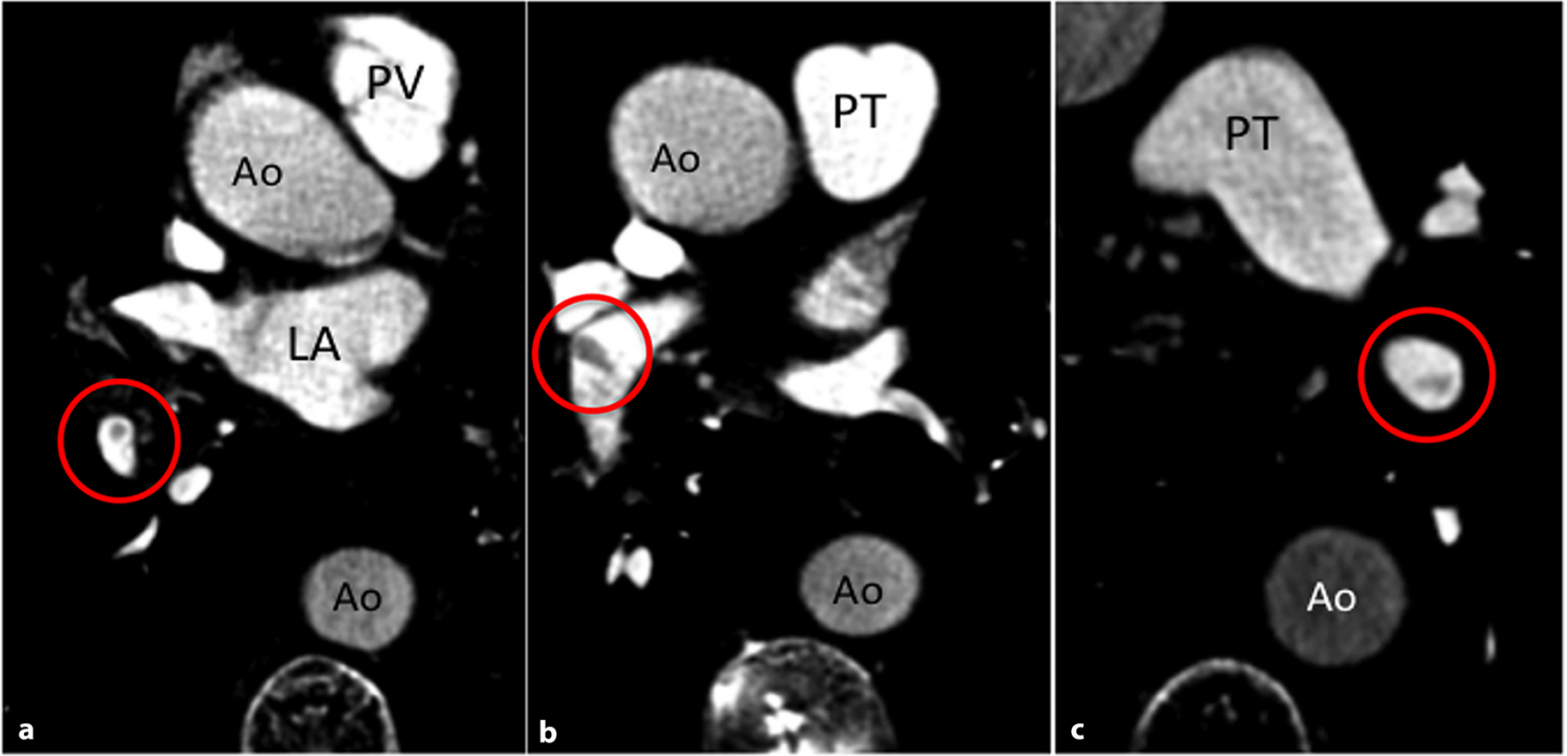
(a–c) CT pulmonary angiogram. Selected axial slices with optimization of windowing for pulmonary arteries. Filling defects are visible within multiple pulmonary arteries (red circles). Ao, aorta; LA, left atrium; PT, pulmonary trunk; PV, pulmonary vein.
